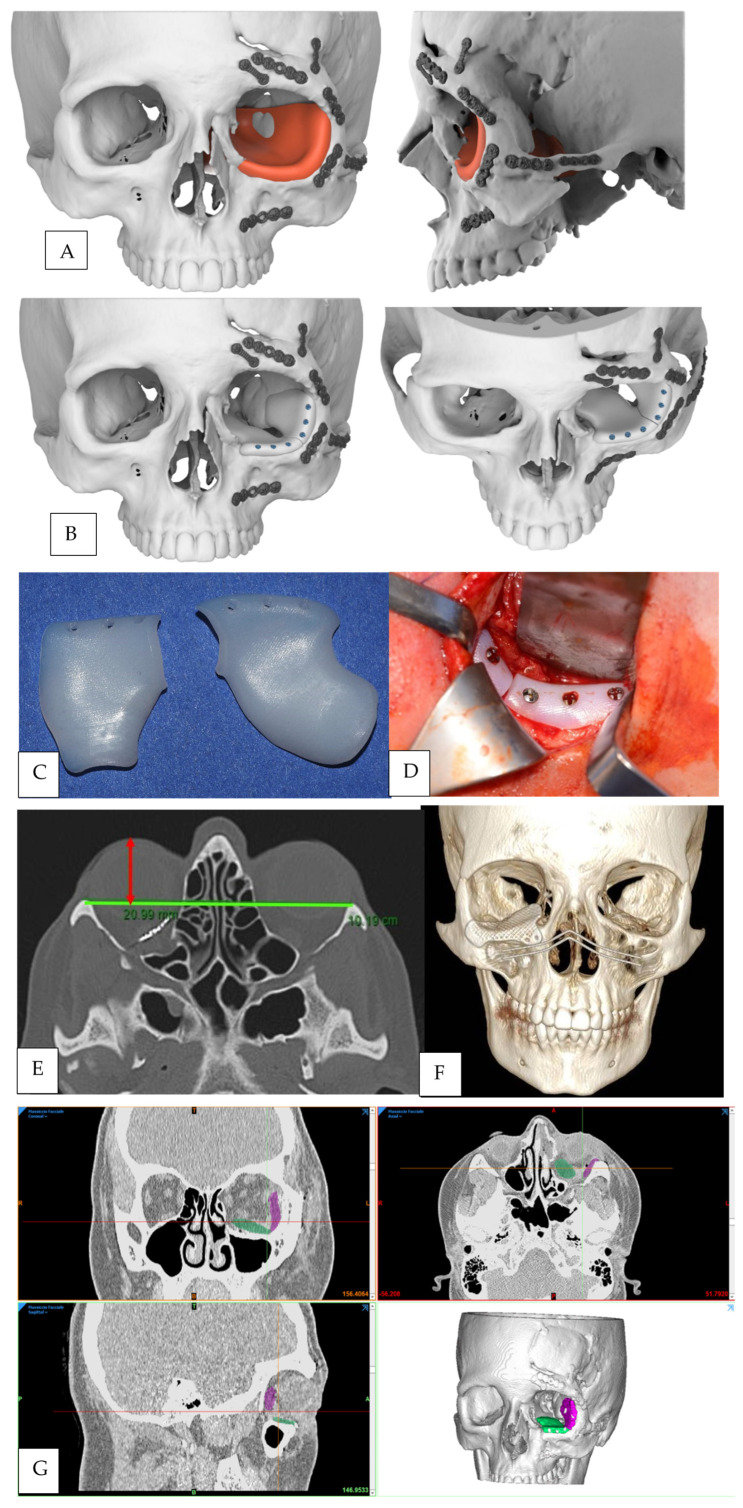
(A) Mirroring of the healthy bone surface on the affected side using 3D reconstruction of the CT scan. (B) CAD of the reconstructive left orbital floor implant. (C) High-density polyethylene implant in two pieces. (D) intraoperative image showing left orbital floor reconstruction via a transconjunctival approach through HDPE implant insertion. (E) Technique of proptosis measurement with CT as proposed by Ramli [18]. (F) Postoperative three-dimensional CT scan showing right orbital floor reconstruction using a customized titanium mesh. (G) 3D segmentation of the postoperative CT scan showing positioning of the HDPE implant with two different colors indicating each component of the prosthesis (green: medial component of the implant, purple: lateral component of the implant).
